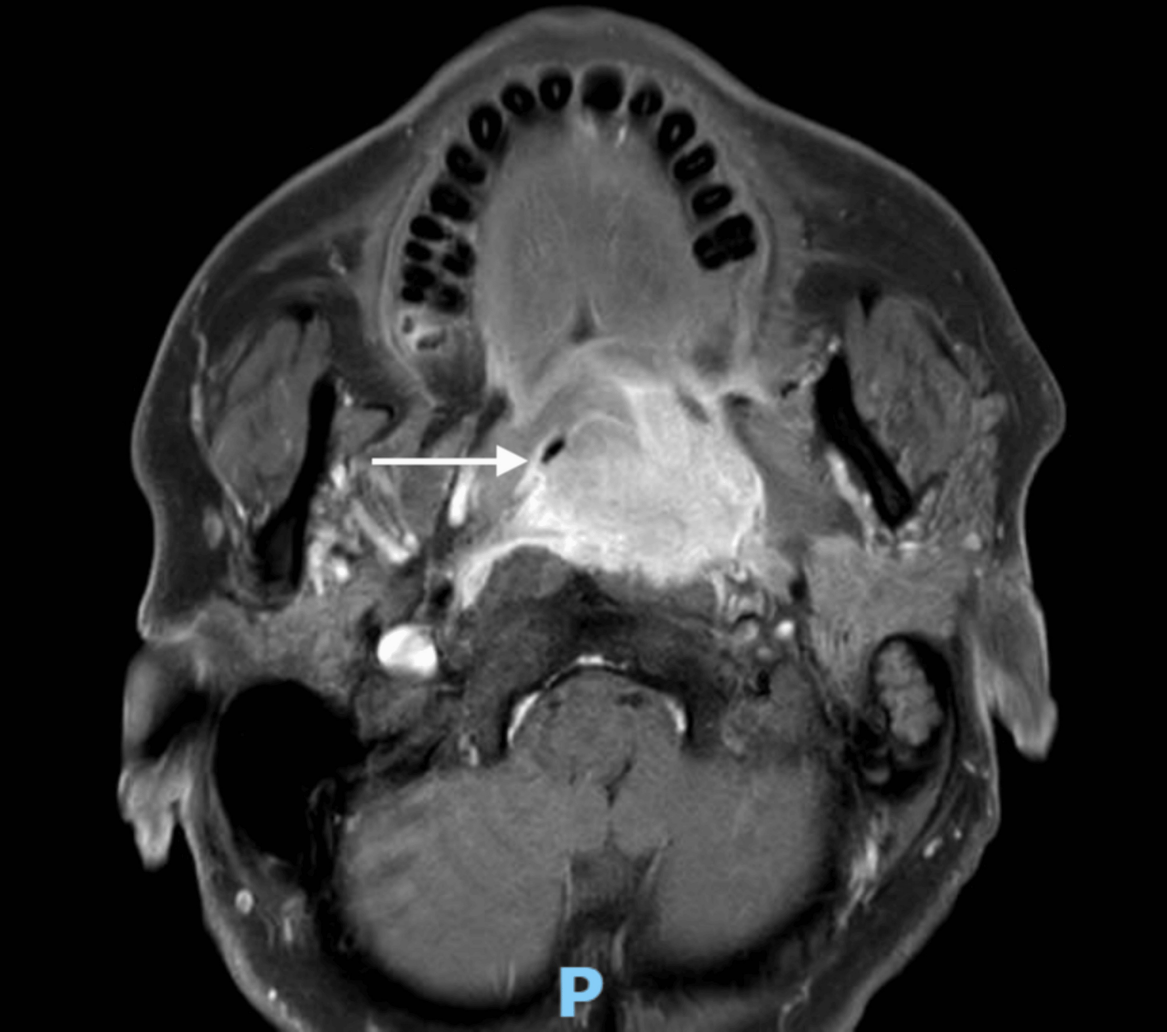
Case 4: MRI neck showing a heterogeneous, enhancing lobulated mass (denoted by white arrow) with lobulated margins, approximately 4.7 cm × 4.2 cm × 3.0 cm, centered at the left tonsillar fossa, with local extension to the tongue base/soft palate, nasopharynx, retropharyngeal/prevertebral, and parapharyngeal spaces.
